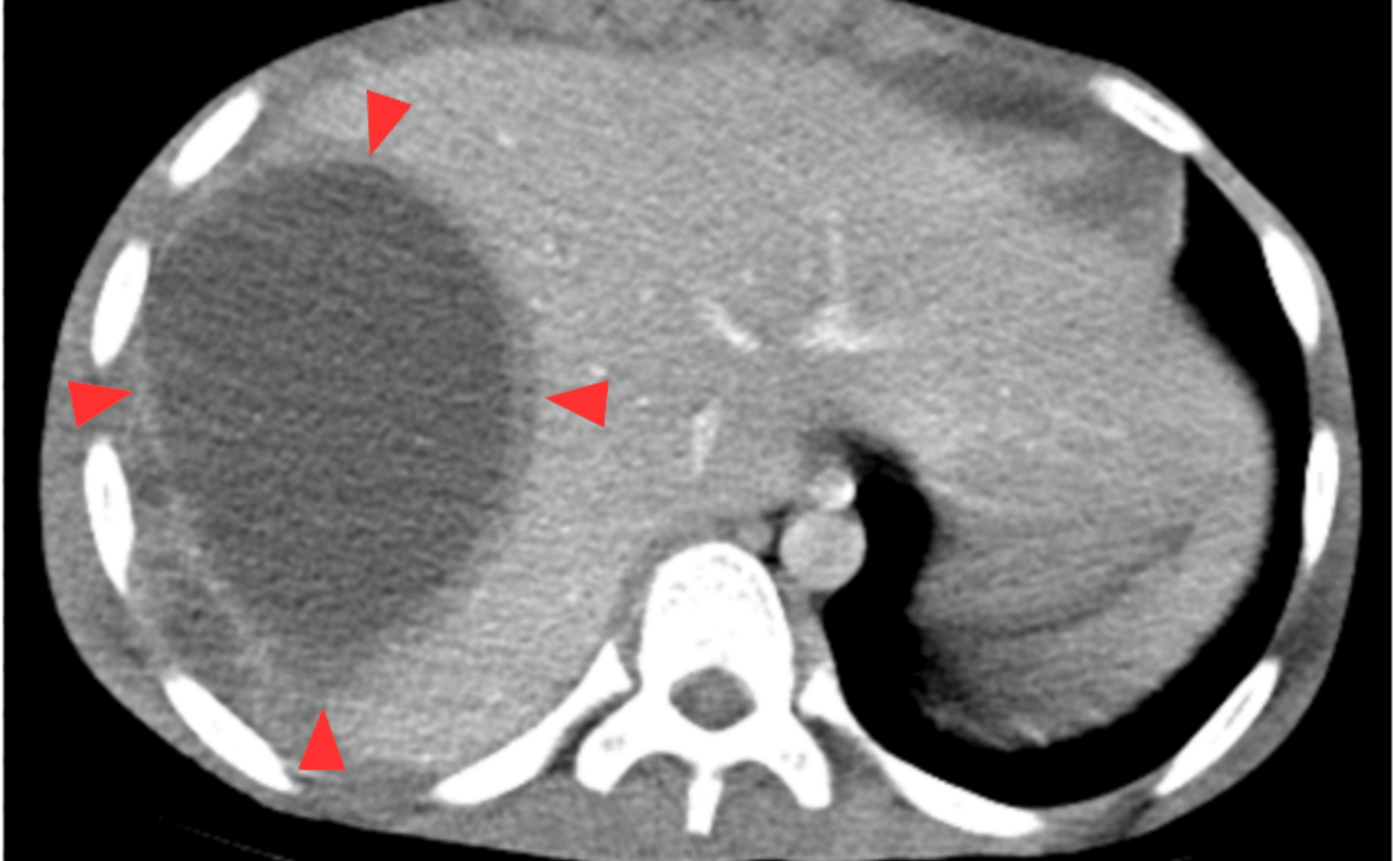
Non-contrast transverse plane CT showing liver abscess measuring 10.1 cm by 9.0 cm by 12.5 cm in the right lobe of the liver with an enhancing wall measuring 6 mm.
